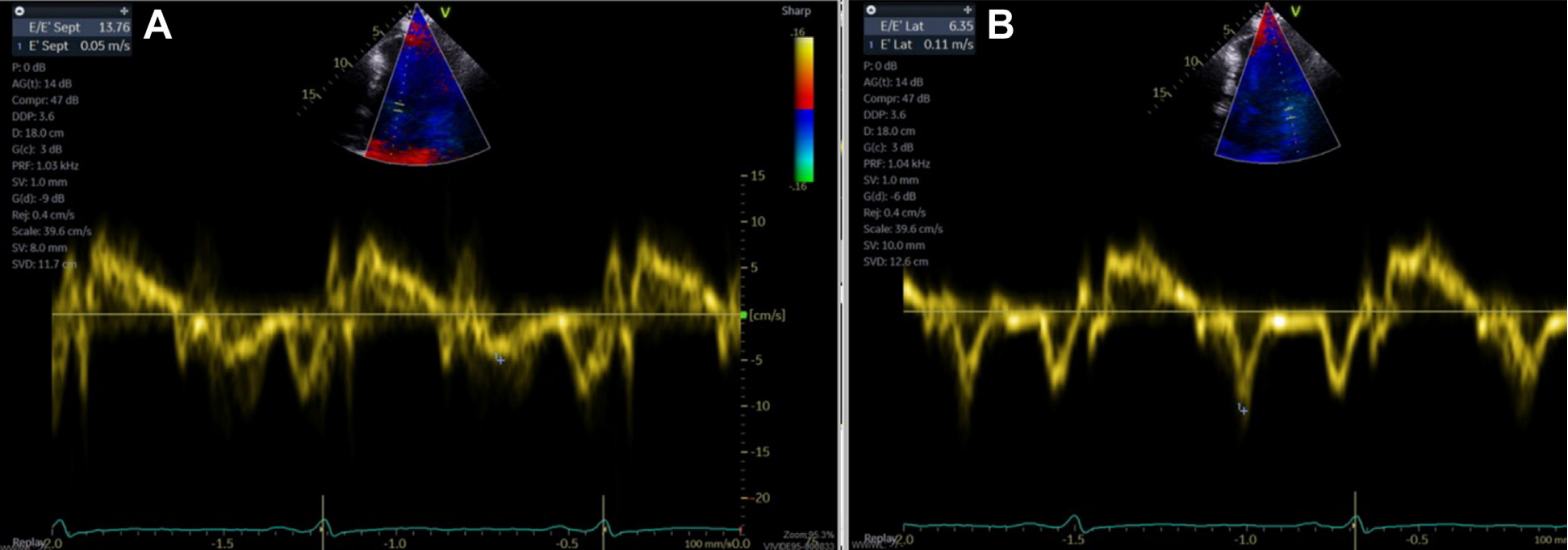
Transthoracic Echocardiogram With Tissue Doppler After Immunosuppressive Therapy Echocardiogram demonstrating normalization of the relationship between medial eʹ and lateral eʹ velocities after immunosuppressive treatment.
